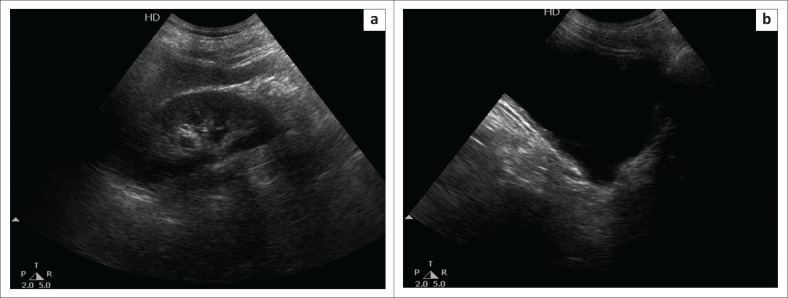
(a) Ultrasound images of the normal right kidney (b) a small diverticulum is present in the region of the right vesicoureteric junction. A normal calibre right ureter is seen opening into it.
